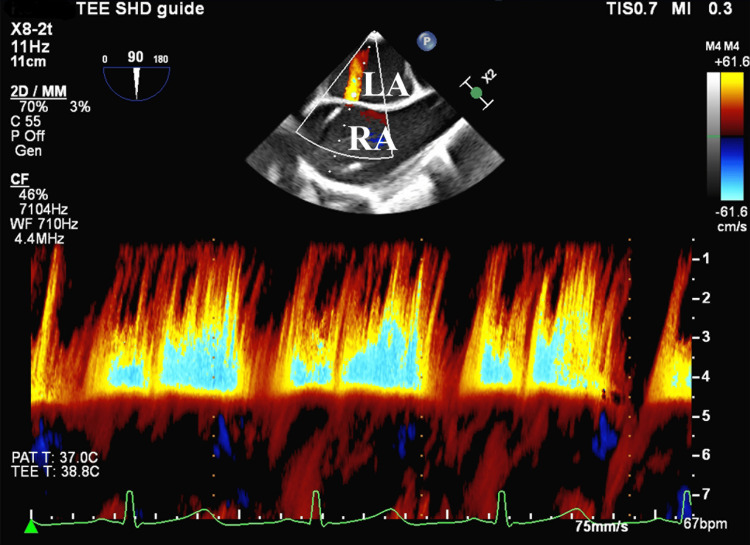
Image of intraoperative screening with transesophageal echocardiography A right-to-left shunt became continuous after removing the steerable guide catheter. LA: left atrium, RA: right atrium
